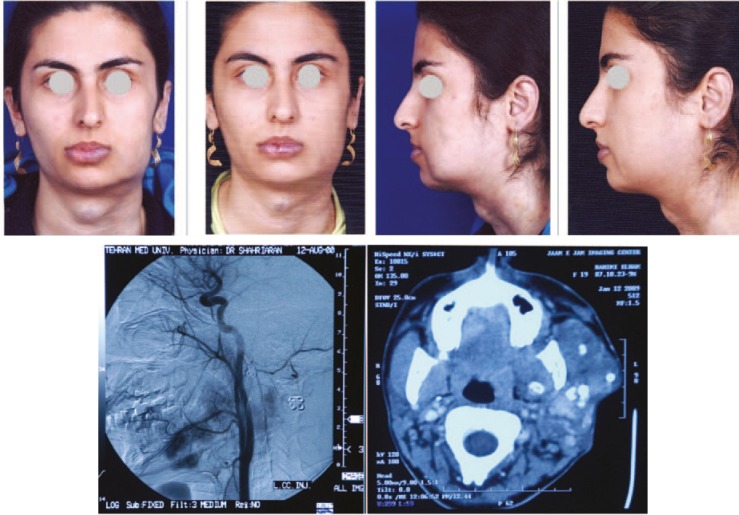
A 30 years old female patient with venous malformation of lower lip, face, neck. She has had 6 injections of combination (bleomycin, triamcinolone and epinephrine), 3 injections with 1 month intervals and 3 injections with 2 months intervals. Tumor blush in arterial phase of DSA is evident and CT Scan demonstrates soft tissue edema and phlebolithes.
